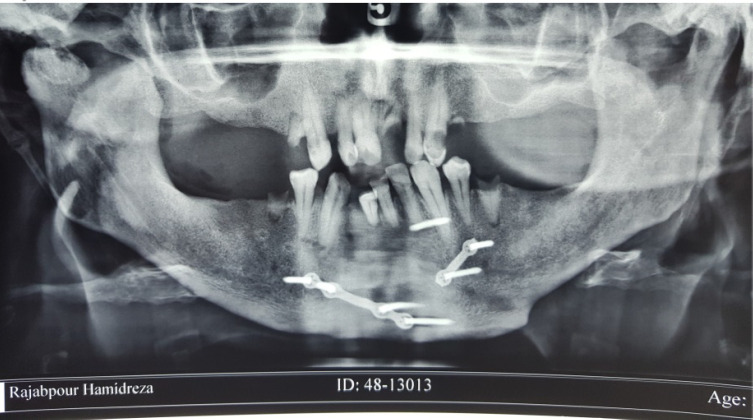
The radiographic view of right TMJ ankylosis
